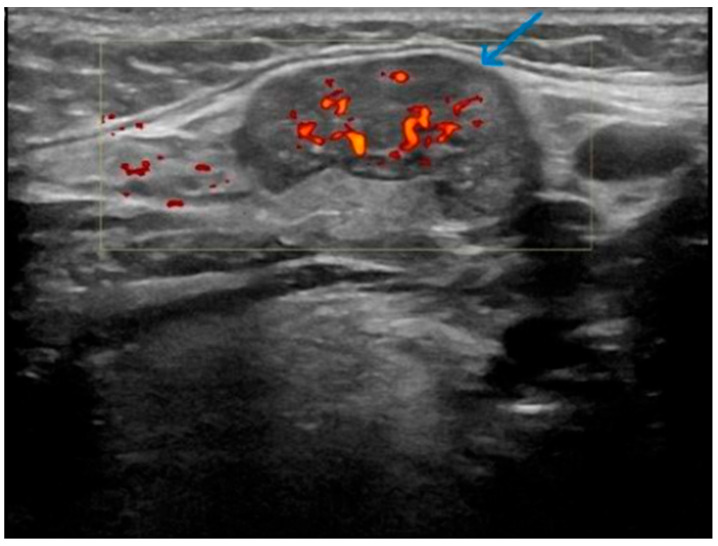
Patient Y—Initial ultrasound scan of affected SMG. Circumscribed focal area of low echogenicity and increased vascularity (indicated by the blue arrow) in the left SMG.
